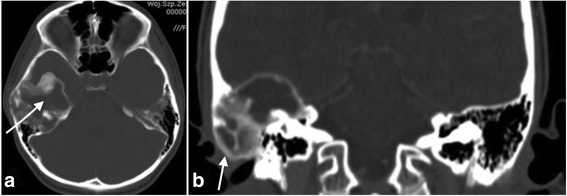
a Axial CT scan shows an expansive lesion of the right petrous apex. b Coronal CT scan demonstrates fibrous dysplasia of the right temporal bone that has led to external deformity
